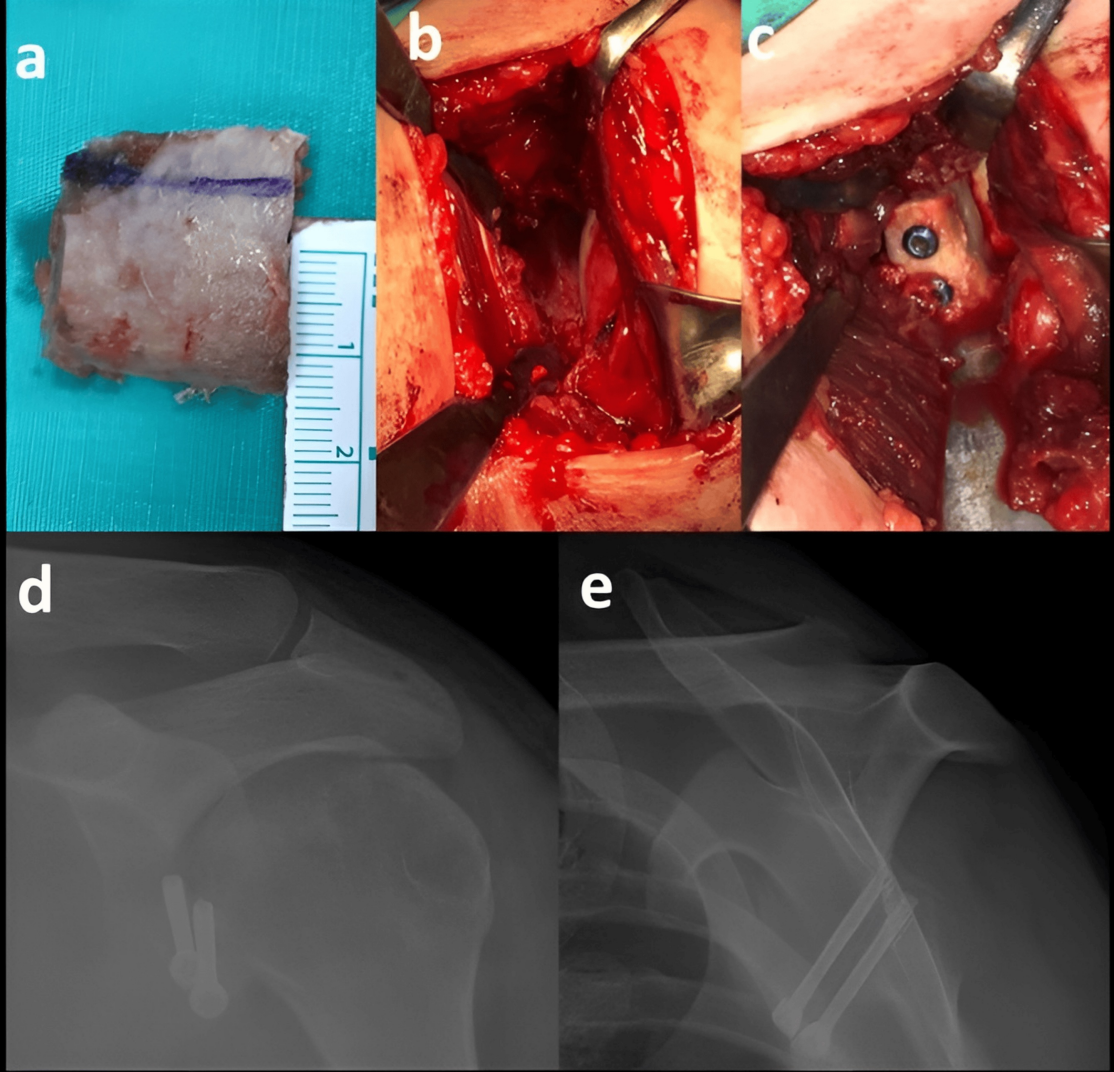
Intraoperative images and postoperative radiographs a) Autogenous bone graft (20 x 15 mm) taken from the iliac crest. b) Preparation of the anterior glenoid rim. c) Fixation of the autogenous bone graft with two 3.5 mm cannulated screws. d-e) Postoperative direct radiographs.
